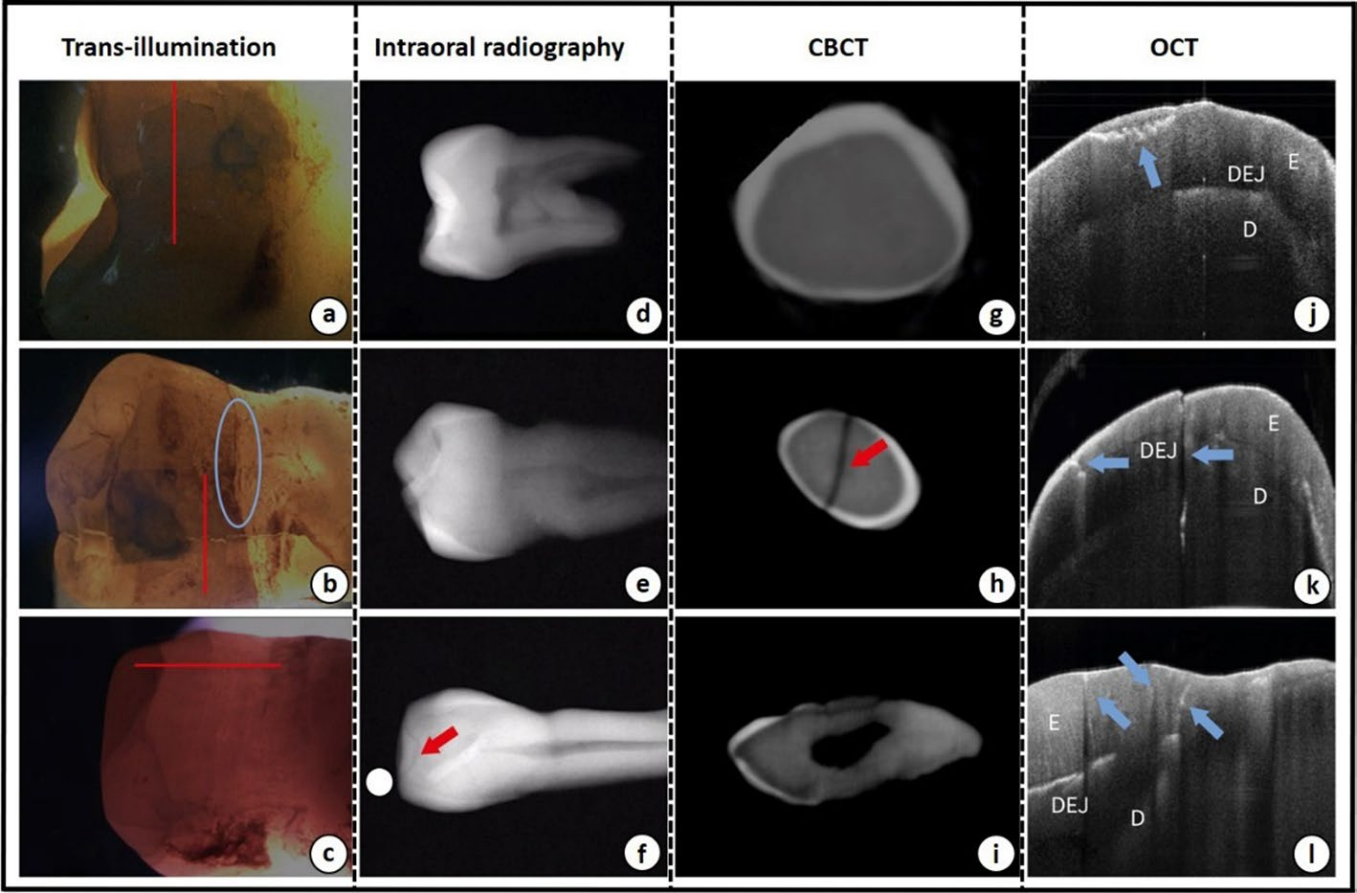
Comparison study of four crack detection methods: (a–c) images from trans-illumination detection; (d–f) images from intraoral radiography; (g–i) images from CBCT; (j–l) images from OCT. The red line indicates the cross section of CBCT and the OCT scan line. Red and blue arrows indicate crack lines. The blue circle indicates a false-positive crack in trans-illumination detection. The image resource was cited from reference [53]. Copyright © 2017. Korean Academy of Periodontology publishing
